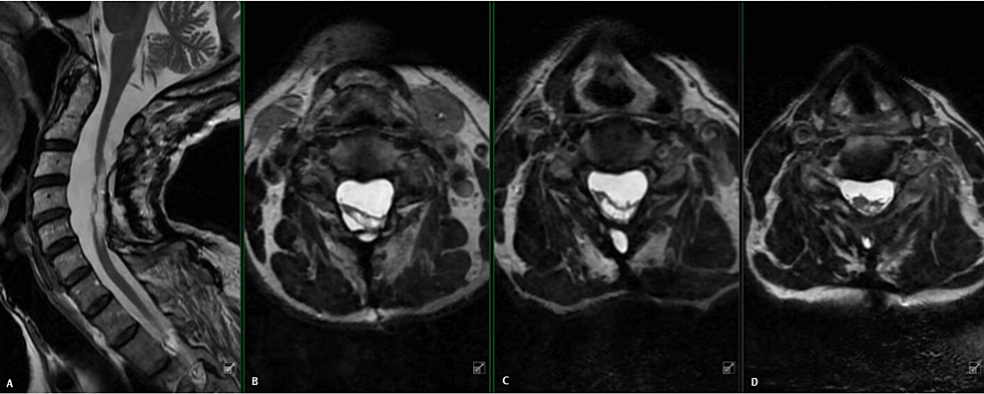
Preoperative MRI of the cervical spine with mid-sagittal view (A) and selected axial T2-weighted images at C3/4 (B), C4/5 (C) and C5/6 (D), demonstrating dorsal tethering of the cervical spinal cord to the overlying dura from approximately C3 to C7. There is eccentricity of the tethering to the left side, as well as a large central syrinx with marked atrophy of the cord.
